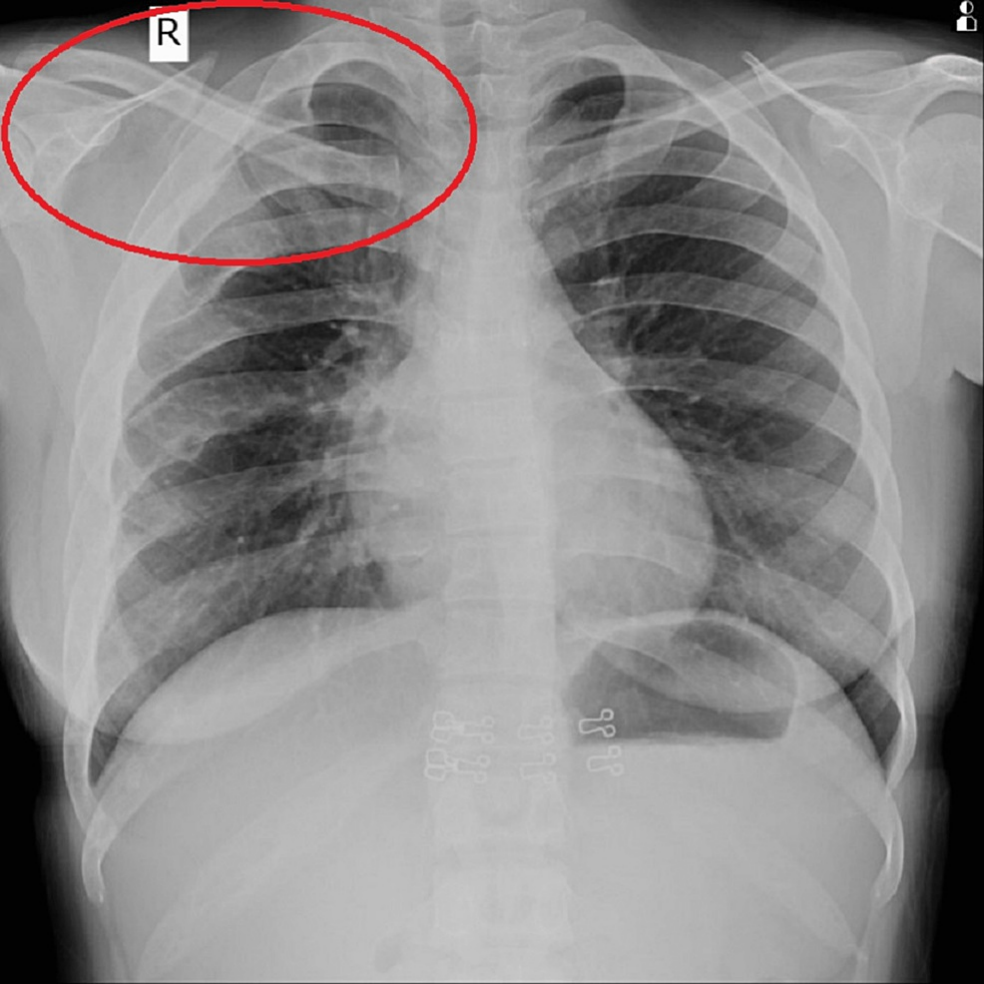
Chest X-ray showing the expansion of right scapula, first and second rib, and sternum
